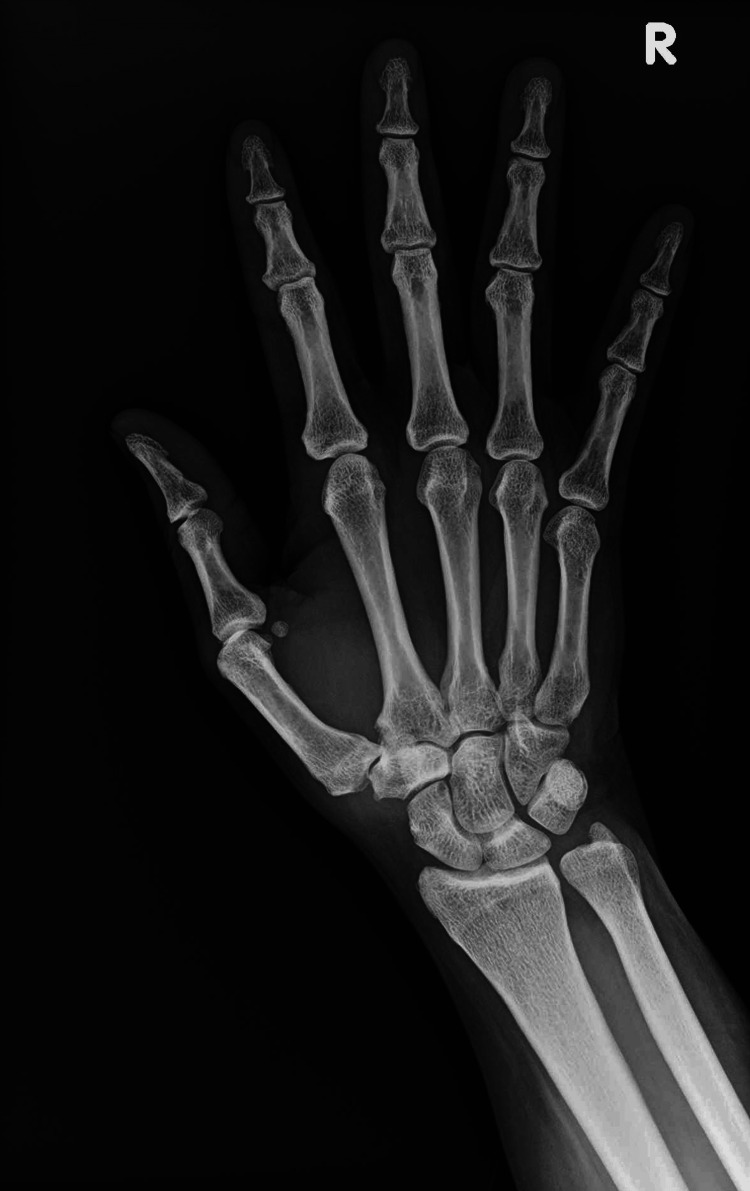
Posteroanterior radiograph of the right hand demonstrating moderate osteoarthritis of the first carpometacarpal joint as evidenced by decreased joint space, osteophytes, and subluxation.
